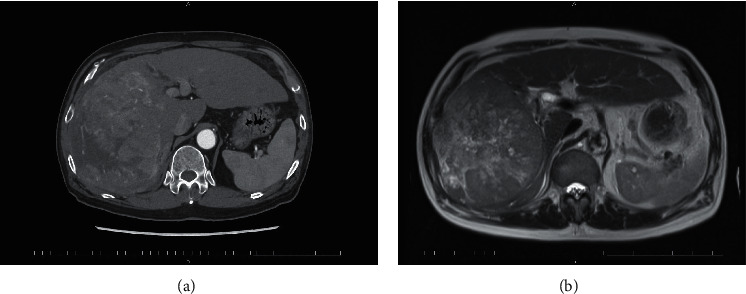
Most of the right lobe of the liver was occupied by a huge tumor (13 cm × 10 cm) on contrast-enhanced CT (a) and contrast-enhanced MRI (b).
